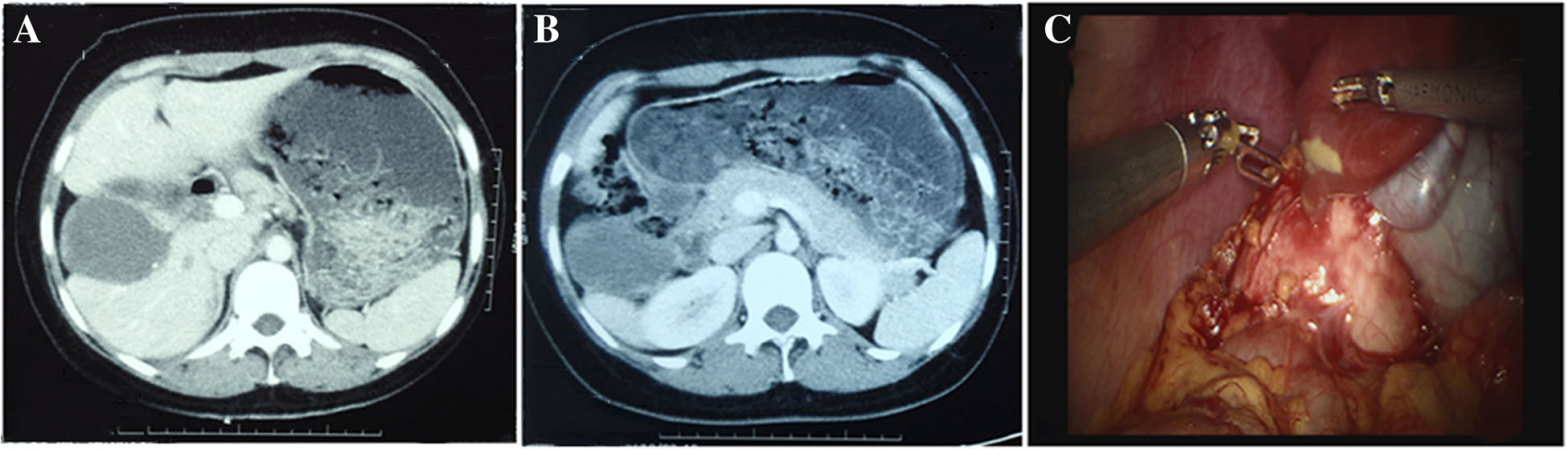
a CT showedabnormal morphology of the right liver, spins to the right; b CT showed a hepatic hydatid cyst located in the anterior segment of the right lobe of the liver; c Intraoperative visual field showed hydatid cysts adjacent to the gallbladder and liver spins to the right
